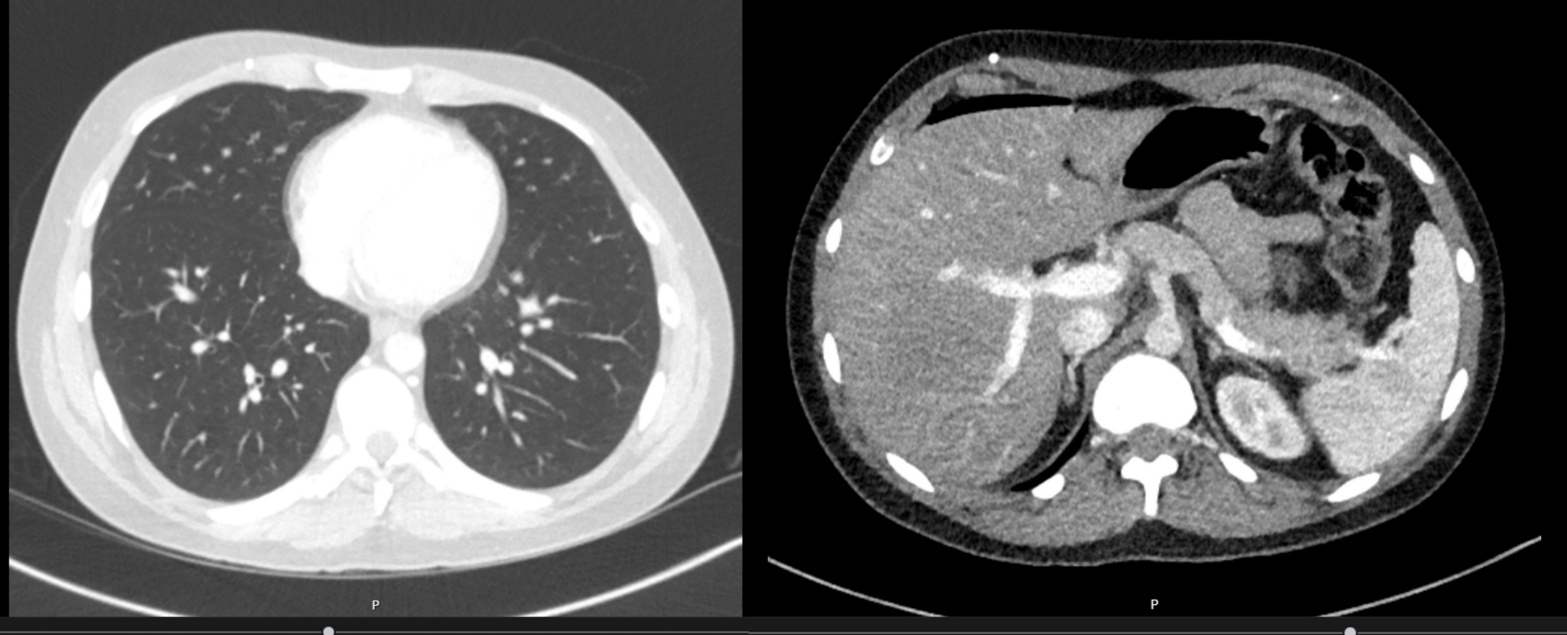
Normal CT of the thorax, abdomen, and pelvis.
